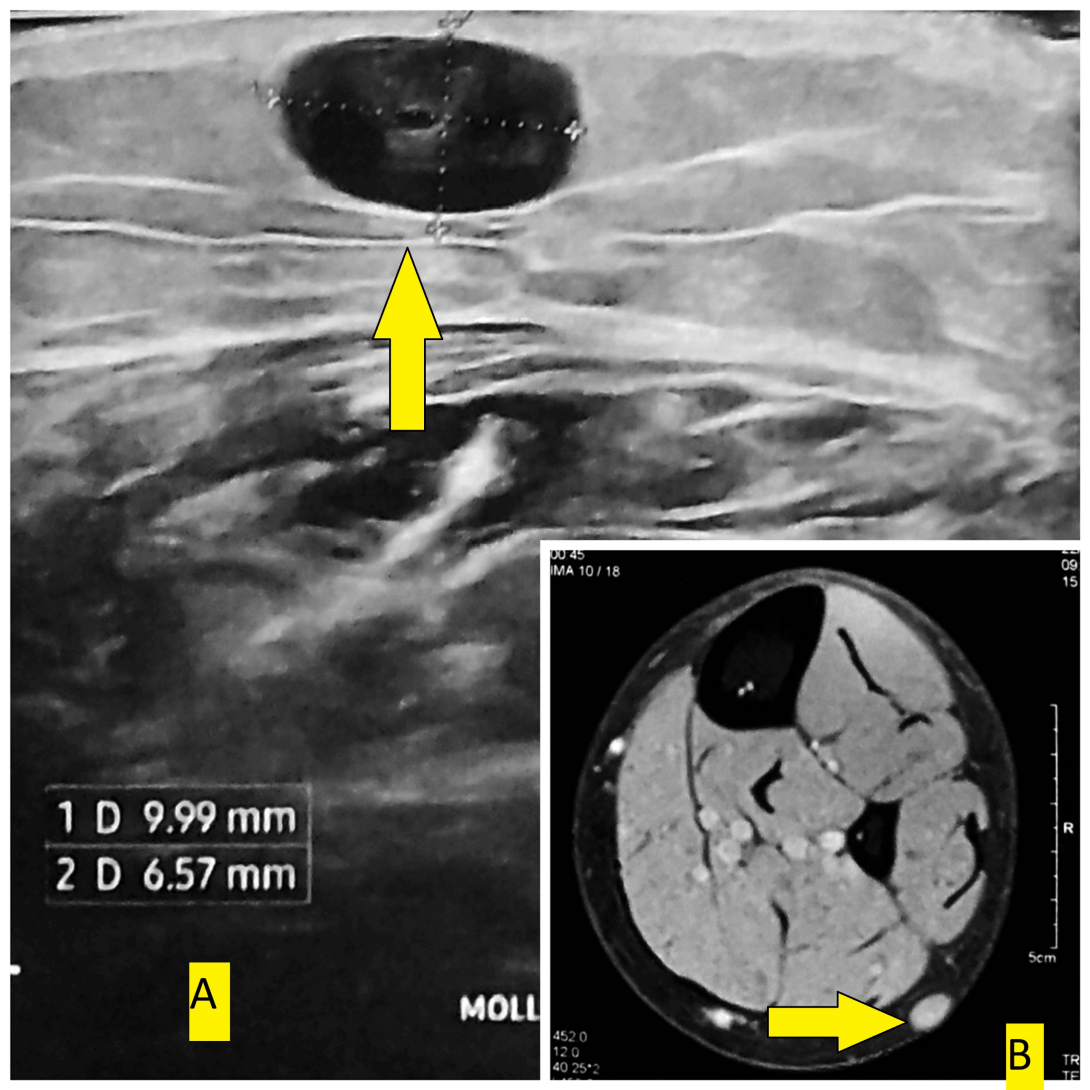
Preoperative scans of the first patient Preoperative echography (A) and MRI (B) images, with arrows marking the mass of interest.
